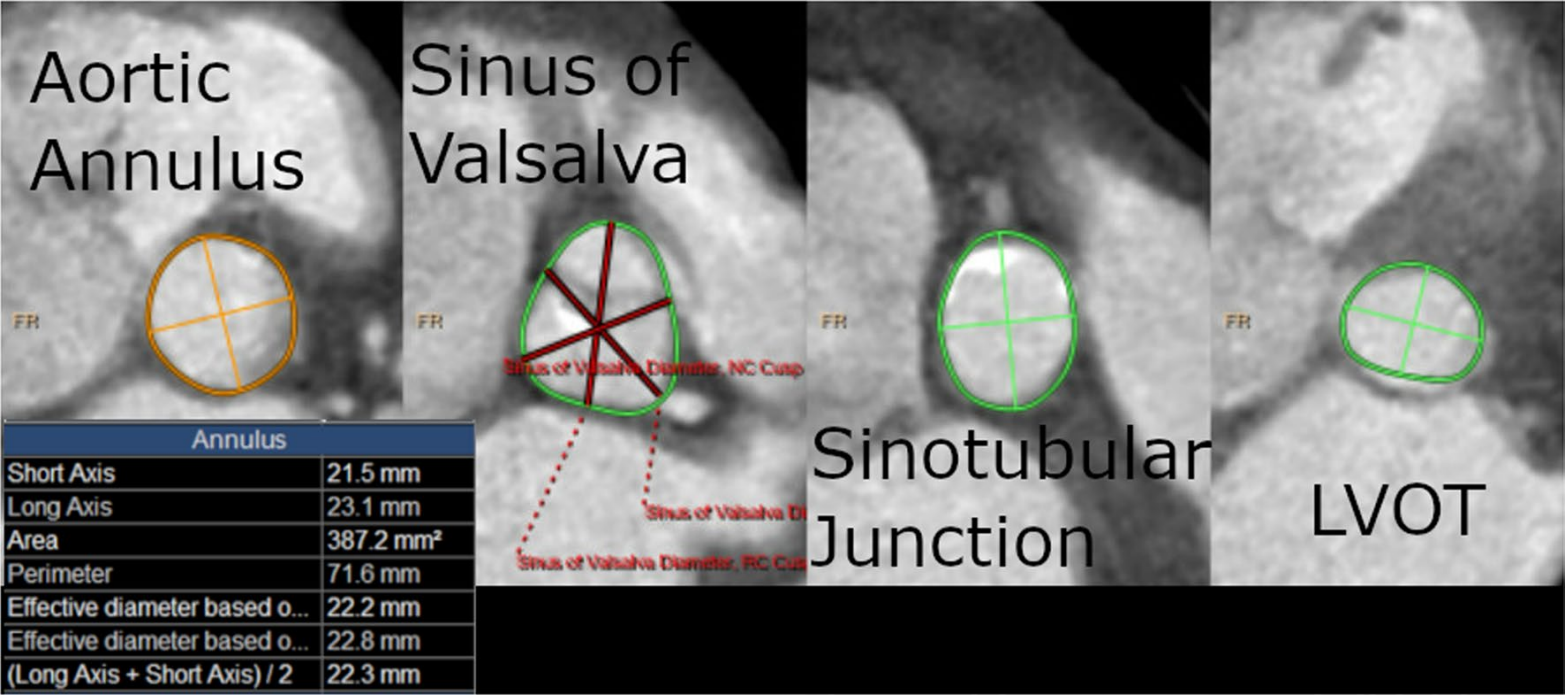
Patient 2 pre-operative CT scan
